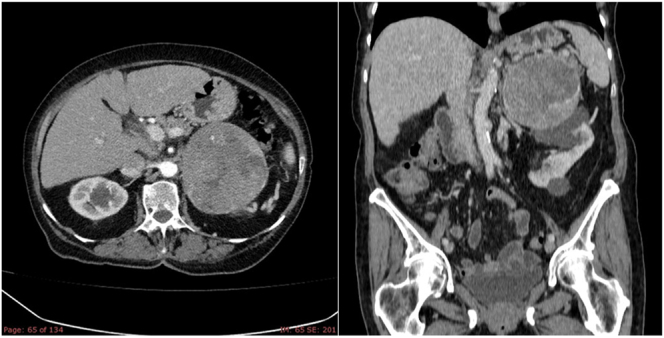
Radiological features of an ACC. CT of the abdomen with intravenous iodinated contrast with abdominal acquisition in arterial and portal phases: solid left adrenal mass with heterogeneous enhancement (8.2 × 9 × 9.3 cm, CCxTxAP). It displaces the tail of the pancreas cranially and caudally to the ipsilateral kidney. It does not show macroscopic fat foci or calcifications. The findings suggest adrenal carcinoma or pheochromocytoma as the first possibility. Pathological retroperitoneal lymphadenopathy (left paraaortic and interaortocaval) and in the hepatic hilum.
